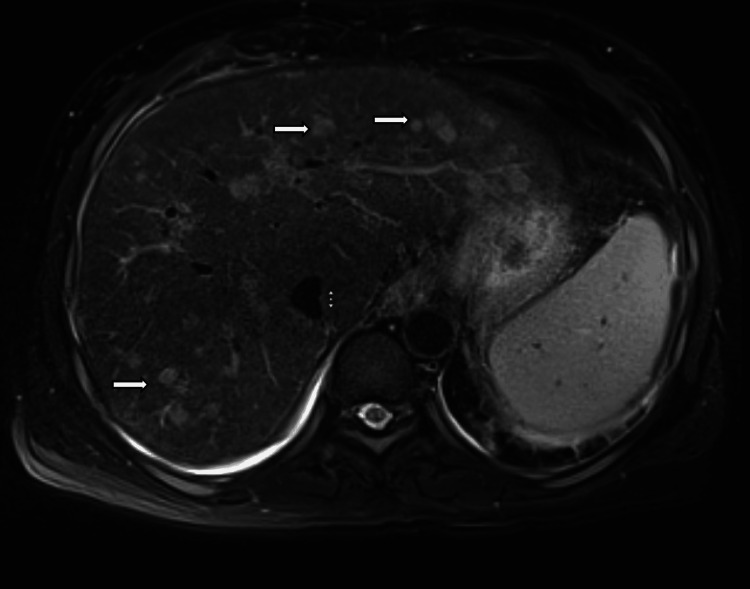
MRI liver with hepatobiliary protocol. Post-contrast axial images show multiple bilobed hepatic lesions with peripheral ring enhancement (arrows), without arterial hyperenhancement or delayed washout.
